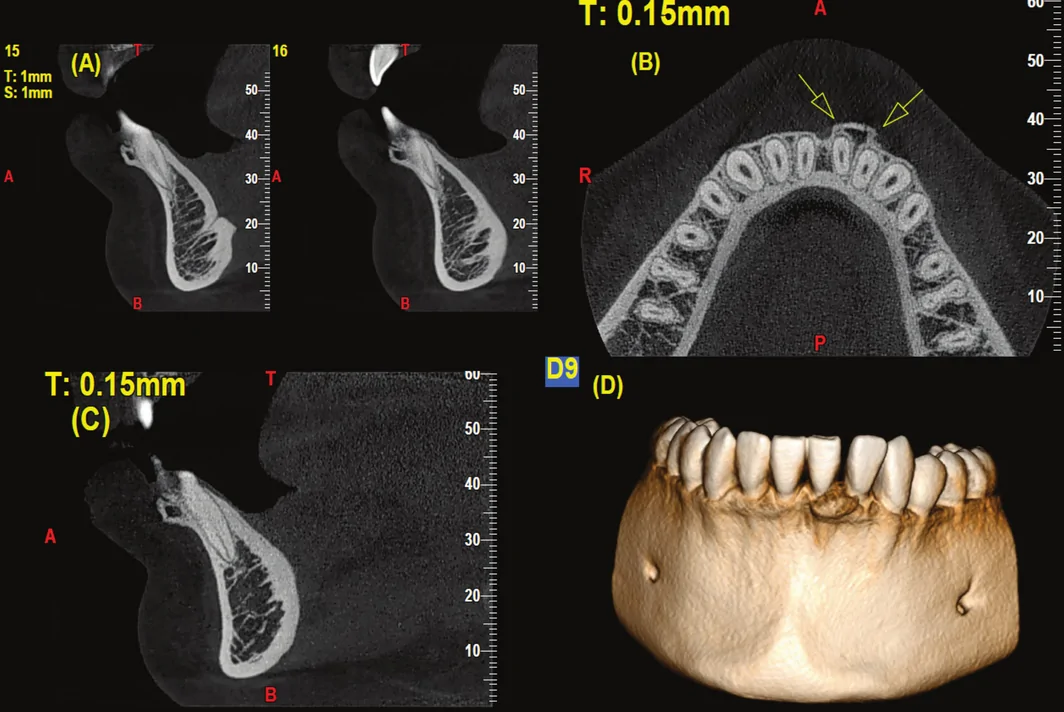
CBCT images show cross‐sectional (A), axial (B), sagittal (C), and three‐dimensional (D) views of exostosis in the anterior region of the mandible.
